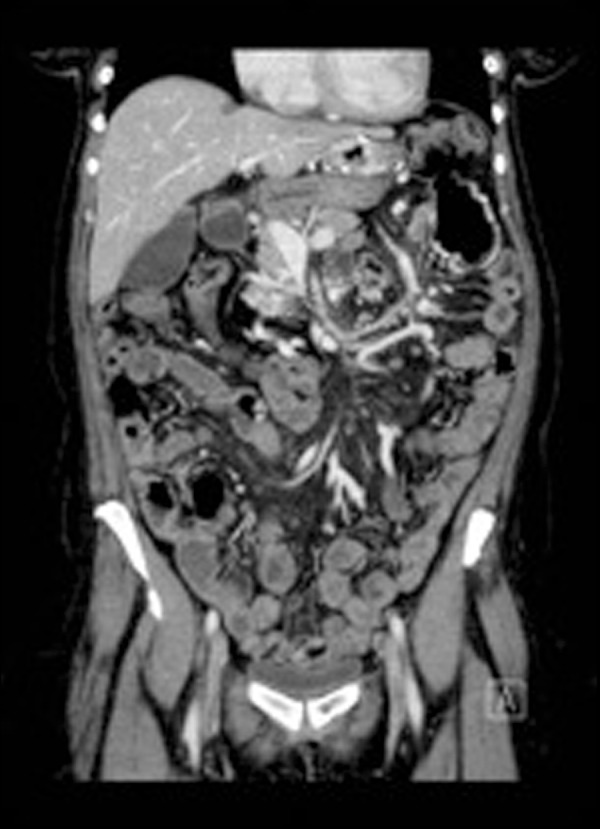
Computed tomography of the abdomen and pelvis: the superior mesenteric vein (SMV) is obliterated, with no visible normal SMV present. Instead, the veins of the small intestine and colon drain into collateral pathways, indicating chronic venous occlusion.
